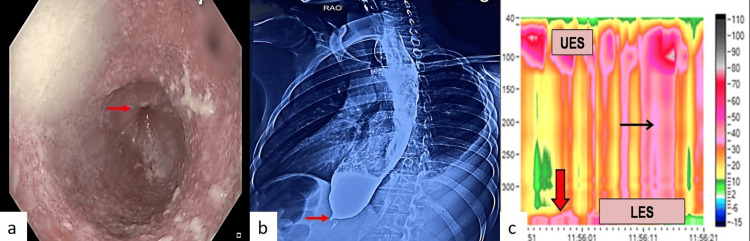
a) Upper gastrointestinal endoscopy showing dilated esophagus with liquid food residue and tight lower esophagus sphincter (red arrow). b) Barium swallow radiograph showing dilated esophagus and narrowing of lower esophagus sphincter (red arrow). c) High-resolution manometry shows increased integrated relaxing pressure (red arrow) and pan-esophageal pressurization (black arrow).
